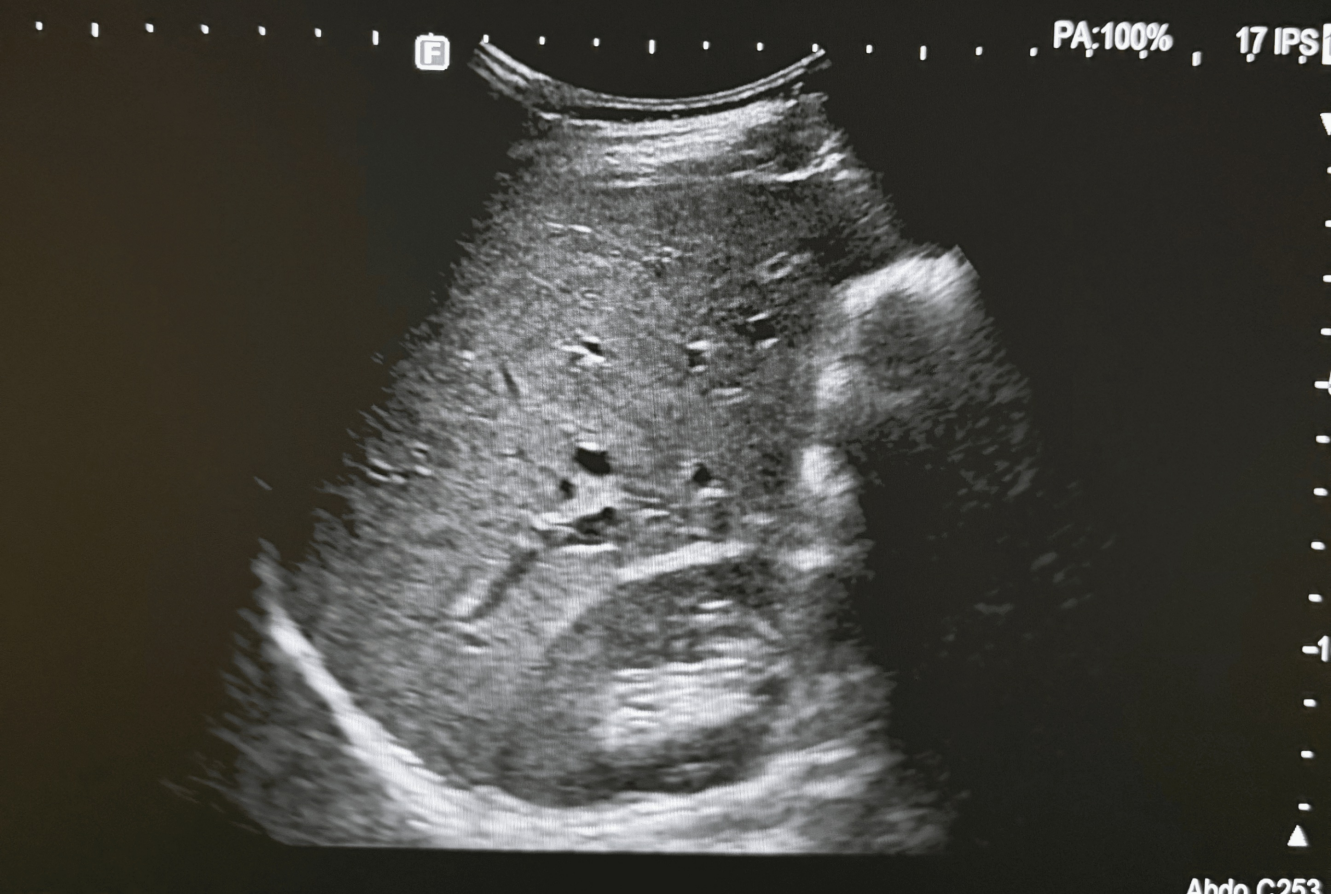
Abdominal ultrasound at 6-month follow-up The ultrasound shows normal liver and kidney appearance.
